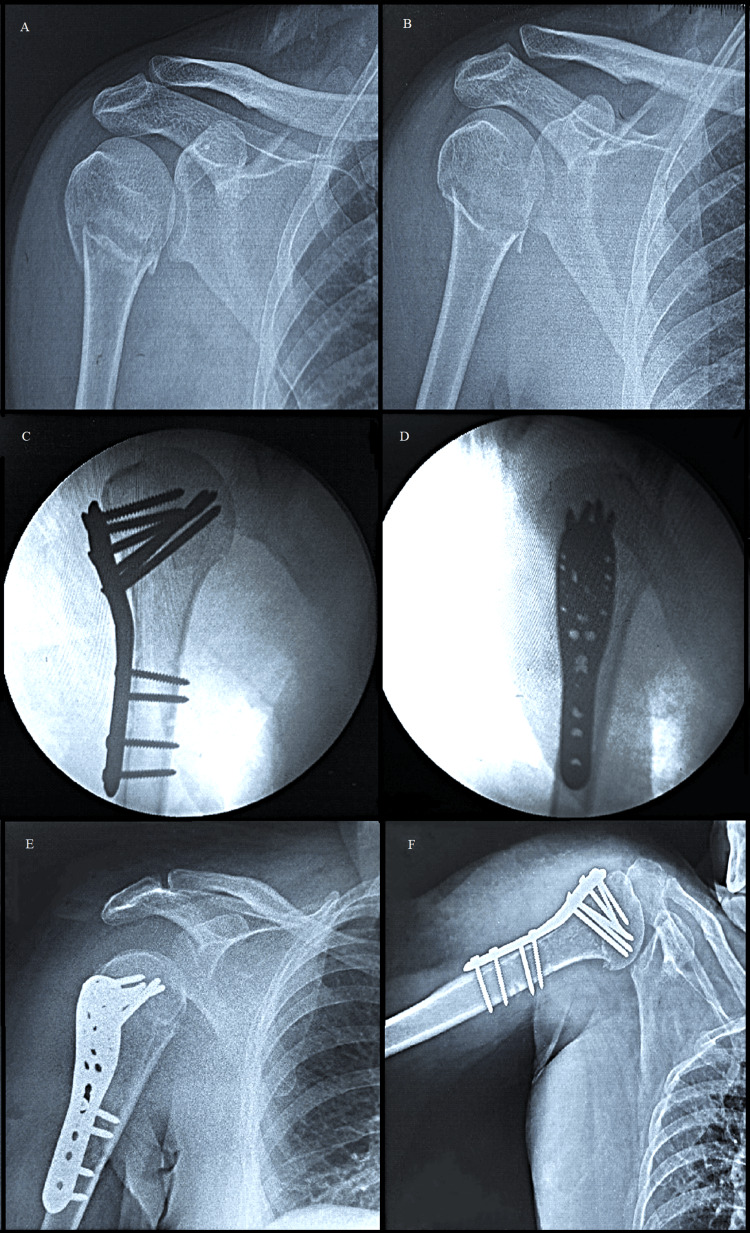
Open reduction and internal fixation of proximal humerus fracture (Neer type 2) with PHILOS plate in a 63-year-old female A: preoperative radiograph of the shoulder in AP view; B: preoperative radiograph of shoulder in the lateral view; C: postoperative C-arm image of shoulder in AP view; D: postoperative C-arm image of shoulder in the lateral view; E: postoperative radiograph of shoulder in the lateral view at the second follow-up; F: postoperative radiograph of shoulder in the axillary view at the second follow-up PHILOS: proximal humerus internal locking system; AP: anteroposterior
